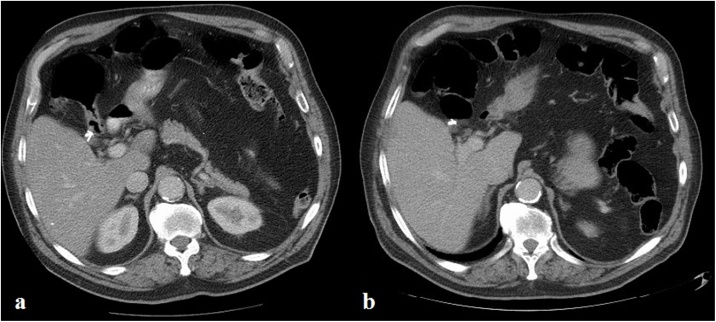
a, b Axial enhanced CT scan demonstrating three endoclips in the hepatic hilum (a) compared with two endoclips in the same location 14 months later (b).
